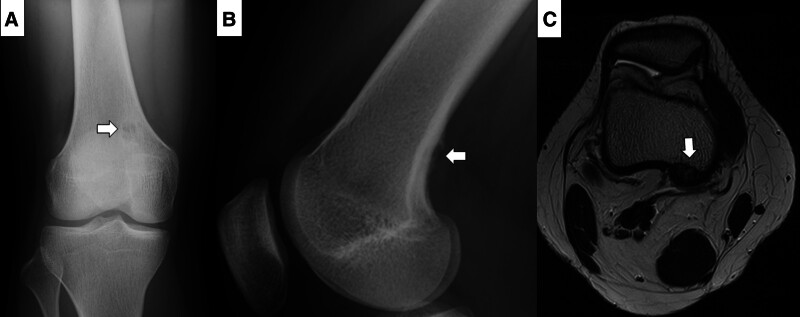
Fifteen-year-old female patient with a suspected osteosarcoma diagnosis made by the primary physician. The anteroposterior (A) and lateral (B) views of the plain radiograph show a small and oval osteolytic lesion with a sclerotic margin (Ritschl stage A). Subtle periosteal reactions are observed. (C) T2-weighted axial MRI confirming no soft-tissue extension from the posterior cortical portion.
